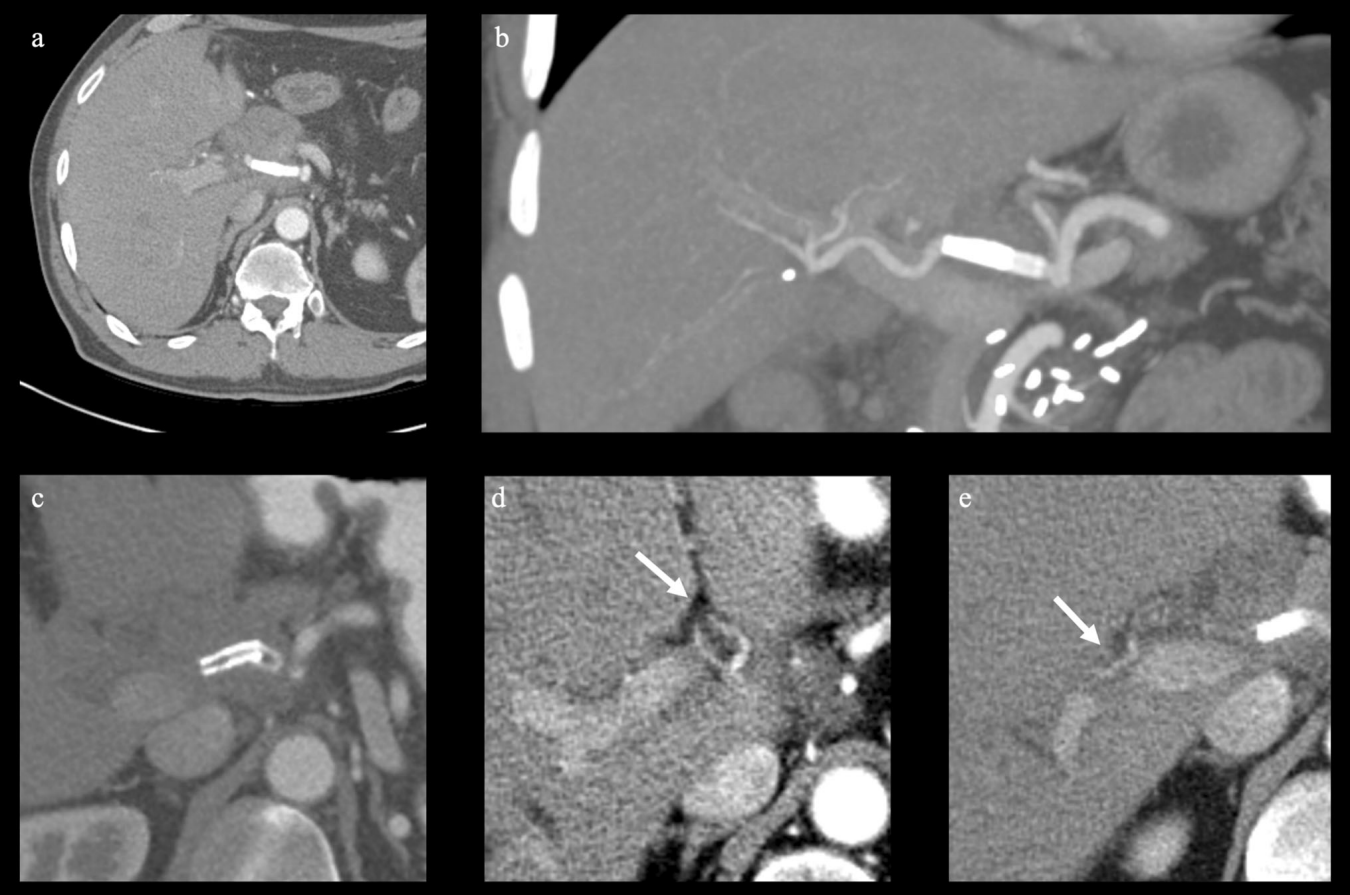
Early follow-up with CT scans in axial reconstruction (a) and coronal maximum intensity projection (b) 20 days after implantation of two stent grafts (proximal 6 × 25 mm, distal 5 × 25 mm VIABAHN®, W. L. Gore & Associates, Inc. Flagstaff, USA) with patency of the stents. Long-term follow-up 468 days after stent implantation showing stent thrombosis (c) with patency of the left (d) and right (e) hepatic arteries (arrows). Images c, d, and e are axial reconstructions
